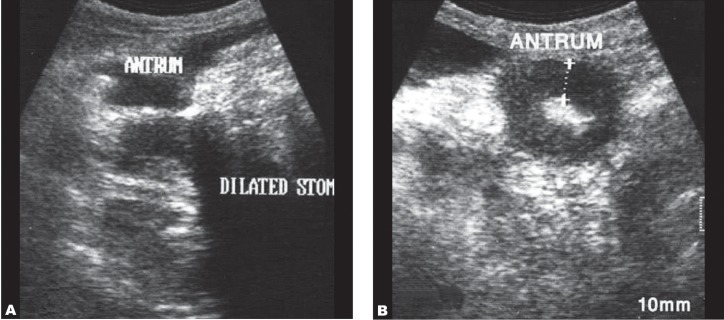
Gastric adenocarcinoma in a 68-year-old man. Longitudinal (A) and transverse (B) abdominal ultrasound images show gastric antrum wall thickening in association with fluid distention in the proximal parts of the stomach.
